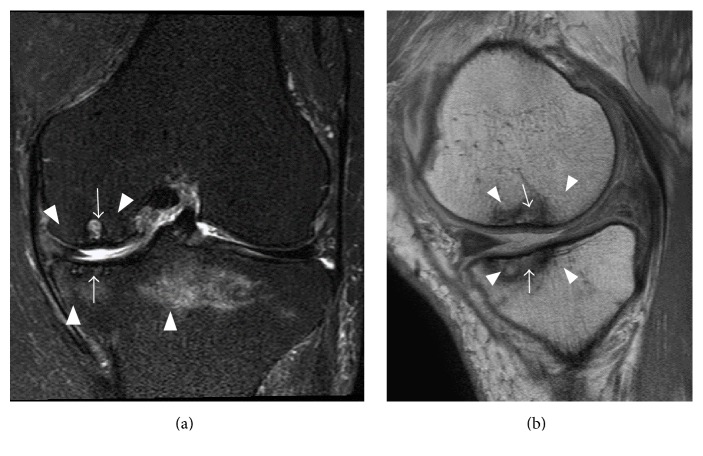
MRI 4 months after first evaluation. (a) Coronal SPIR and (b) sagittal proton density-weighted images showed persistent focal SPONK lesions of both the MFC and the MTP, but the surrounding bone edema had decreased (arrow).
